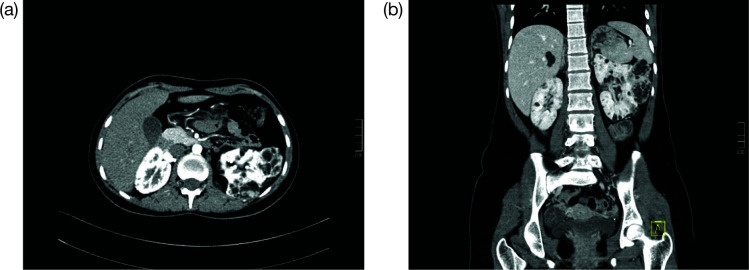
Contrast enhanced CT images of abdomen in axial (a) and coronal (b) planes. This is the case of a 16-year-old female with tuberous sclerosis undergoing treatment with everolimus. The CT scan shows right heminephrectomy and an enlarged left kidney with multiple cysts and angiomyolipomas.
